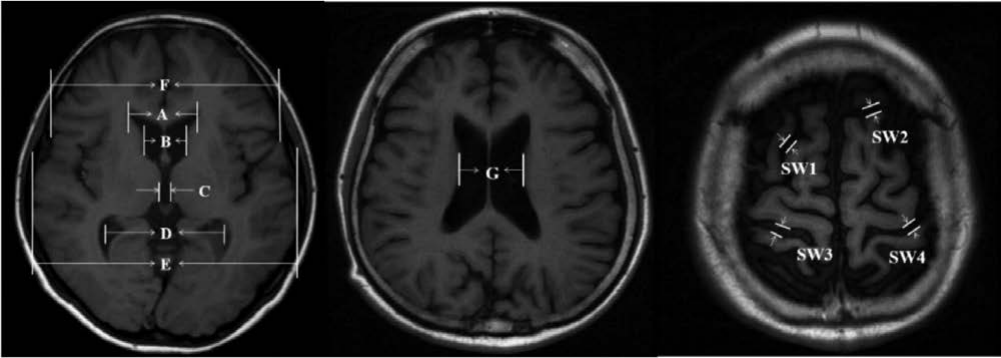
Linear measurements in the brain T1-weighted MRI. A = frontal horns greatest distance, B = minimum distance of caudate nucleus head, C = widest transverse diameter of the third ventricle, D = the choroid plexuses distance, E = maximum internal transverse diameter of skull, F = frontal bones’ greatest external distance, G = maximum external diameter between the lateral ventricular bodies, SW = the four widest sulci at the skull vault.
